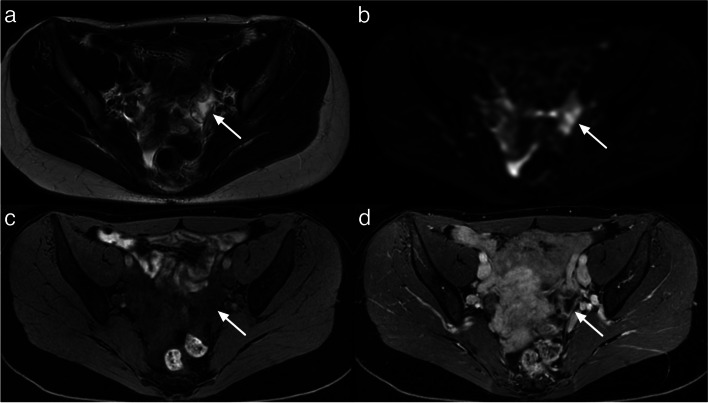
Example of MRI appearance of a corpus luteum cyst. a Axial T2-weighted sequence. b Axial DWI abdominal and pelvic scan (*b*: 1200 s/mm^2^). c Axial unenhanced T1-weighted water sequence. d Axial late-gadolinium T1-weighted sequence. Left ovarian corpus luteum cyst (maximal diameter: 35 mm) (white arrow)
